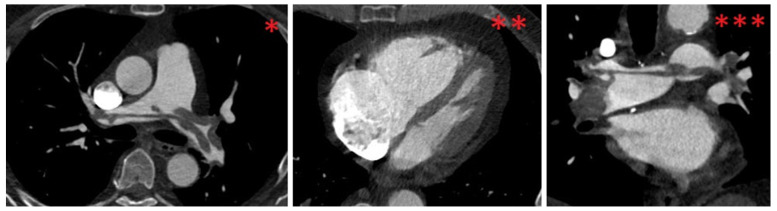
CTPA findings at time of diagnosis. * and : axial view. *: coronal view; bilateral, central saddle pulmonary embolism (*, *); pulmonary artery-to-aorta diameter ratio > 1 (1.1, *); caval contrast reflux (); D-sign (); right ventricle-to-left ventricle diameter ratio > 1 (2.2, ); CTPA, computed tomographic pulmonary angiography.
